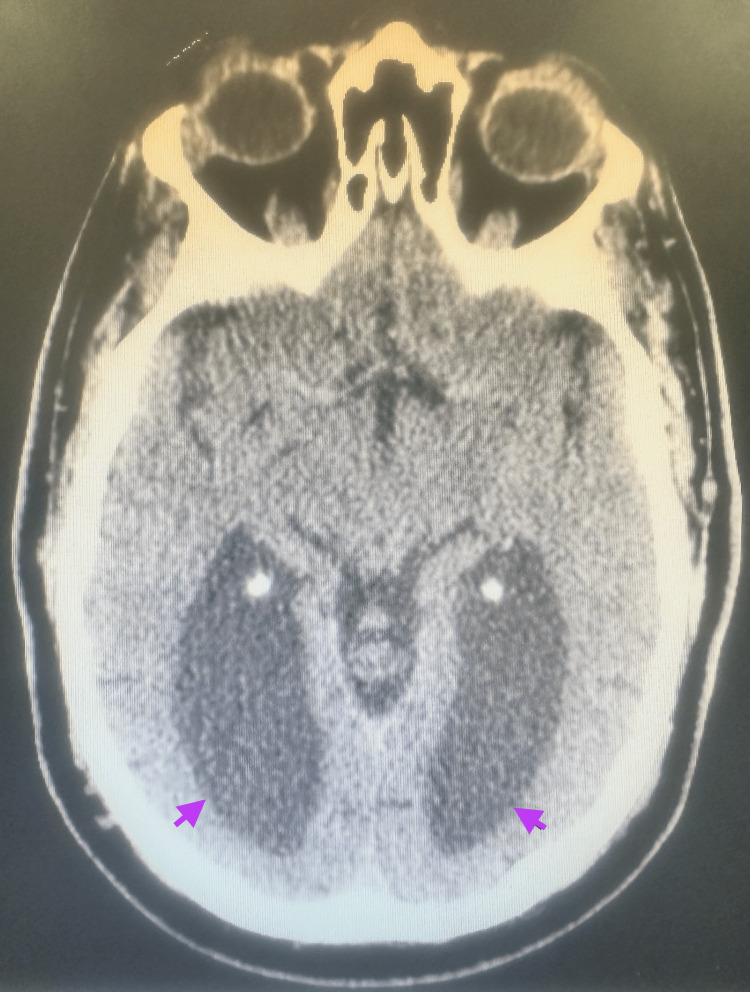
CT showing enlarged ventricles.
